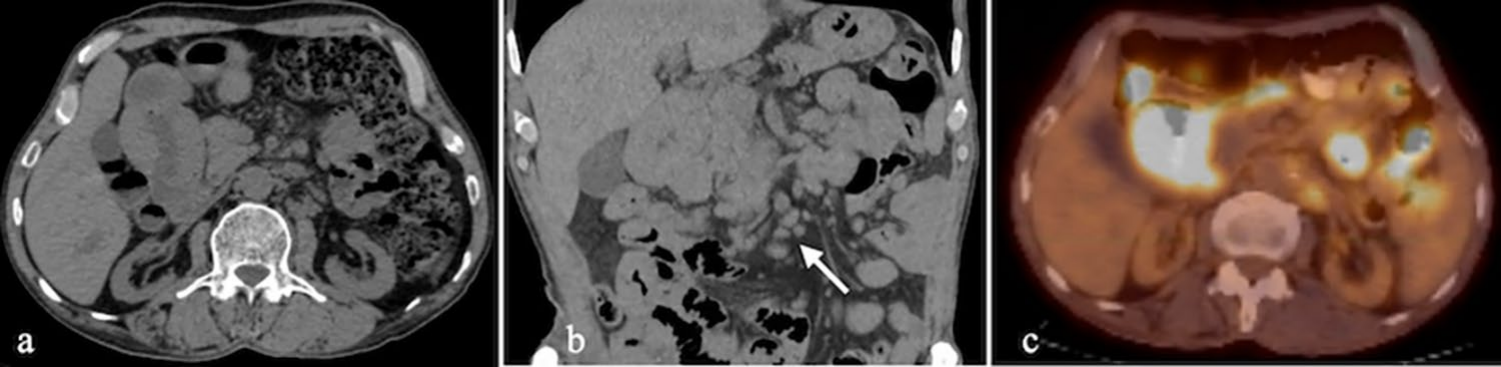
Non-enhanced CT a. Axial, b. Coronal, c. FDG-PET CT fusion images of a 22-year-old male patient diagnosed as T-cell lymphoma. There is a diffuse segmental wall thickening and prominent FDG uptake compatible with lymphoma involvement of the second—(D2) segment of duodenum. Additionally, there are spheric mesenteric lymph nodes (Arrow)
